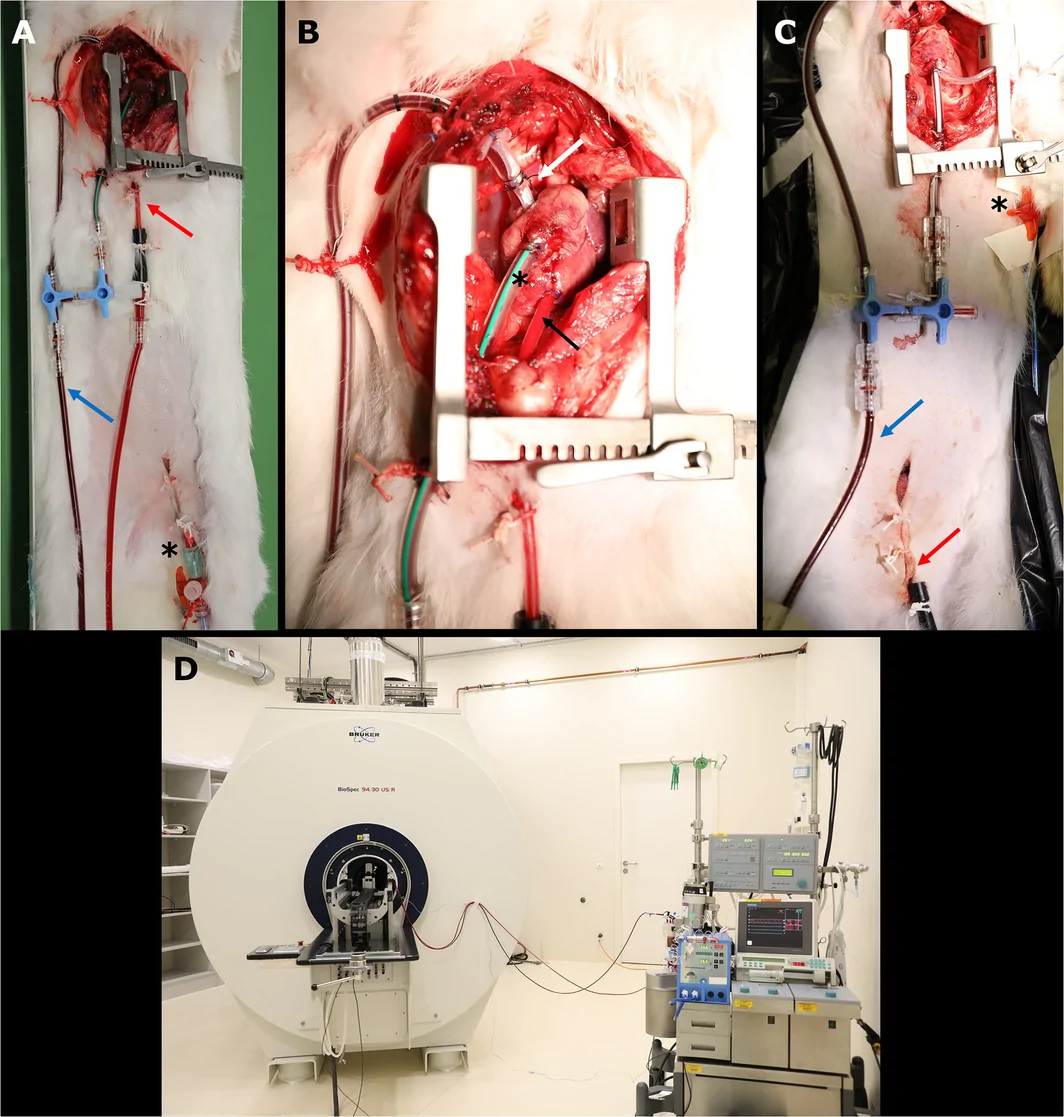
Experimental Setup of ECC installation and MRI transfer. (A) Operative setting for antegrade perfusion: arterial cannula (red arrow), venous drainage (blue arrow), cannula in the left femoral artery for invasive arterial pressure measurement (*). (B) Details of thoracic cannulation: venous cannula in the right atrium (white arrow), venous cannula in the right ventricle (*), arterial cannula (black arrow). (C) Operative setting for retrograde perfusion: cannula in the left brachial artery for invasive blood pressure measurement (*), venous drainage (blue arrow), arterial cannula (red arrow). (D) Setup during MRI measurement: magnetic resonance scanner (left side), heart‐lung machine (right side). [Color figure can be viewed at wileyonlinelibrary.com]
